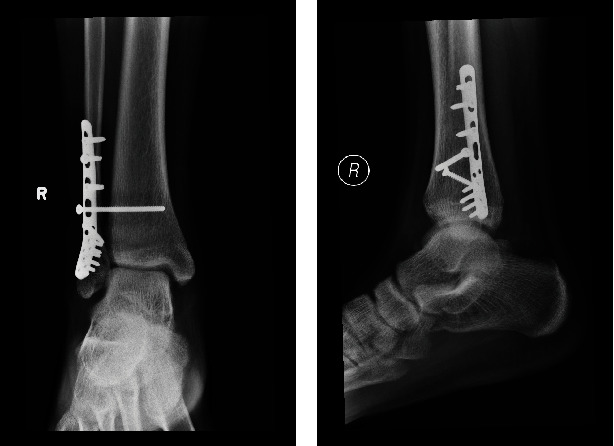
(a) X-ray of the right ankle in anterior-posterior view after operation. (b) X-ray of the right ankle in lateral view after operation.
